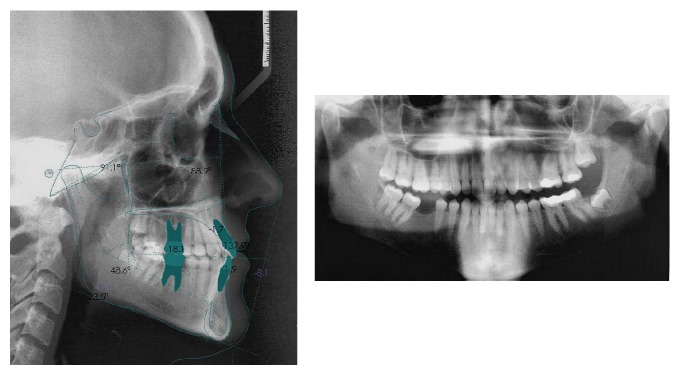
Lateral and panoramic initial radiographies.
